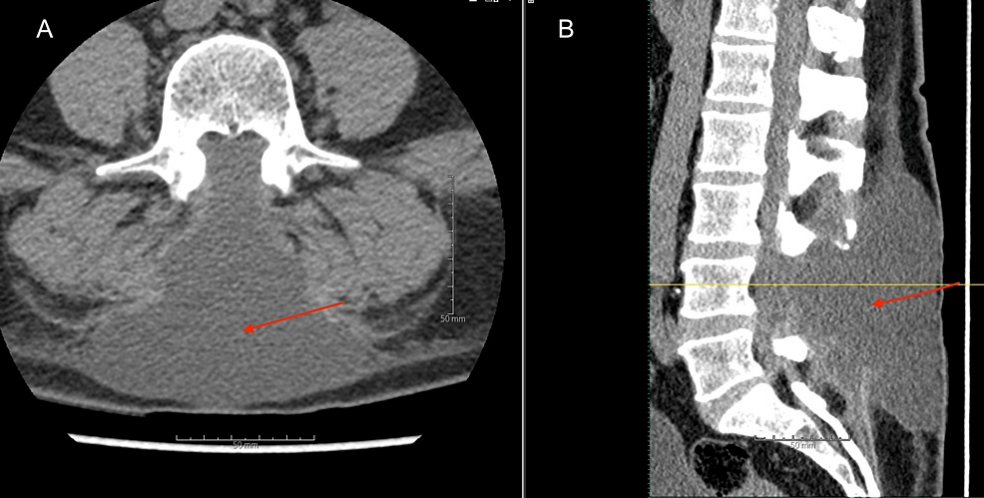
CT of the Lumbar Spine Without Contrast CT scan without contrast of the lumbar spine in the axial (A) and sagittal (B) planes showing a large, non-contrast-enhancing CSF intensity fluid collection within the soft tissue overlying the lumbar spine CSF: cerebrospinal fluid
